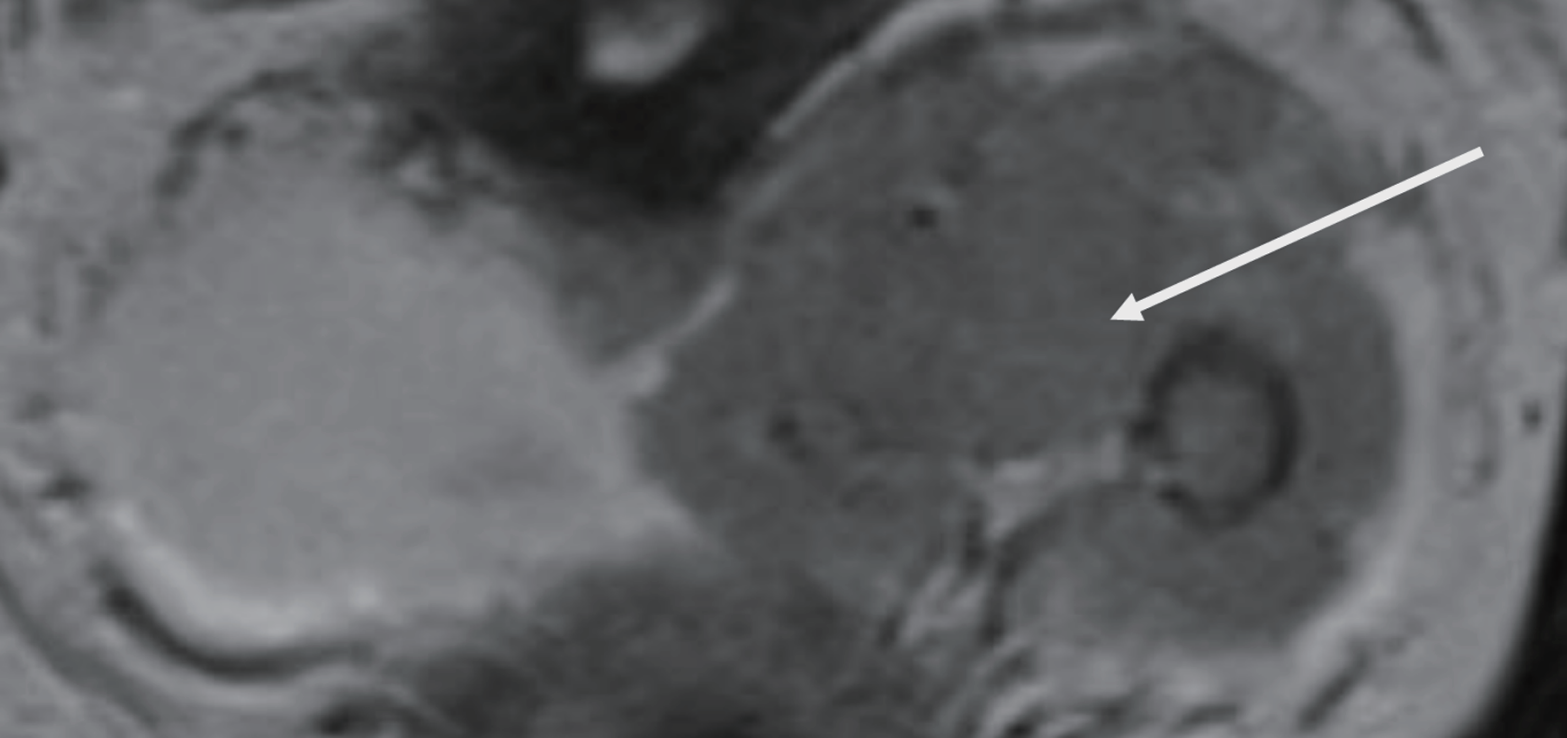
Lower abdomen magnetic resonance imaging (MRI) examination demonstrated tumor invading ureter (arrow).
